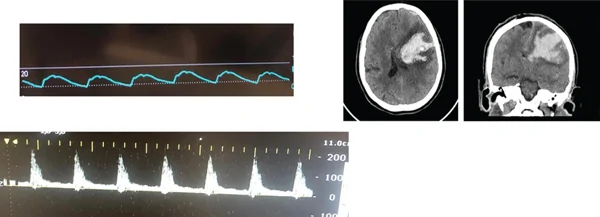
ICP waveform (blue line) with inversion of the p2/p1 ratio, decrease of diastolic flow, and pulsatility index > 1.4 (TCCD) at 24 h. A new CT scan was performed that revealed perilesional edema.
